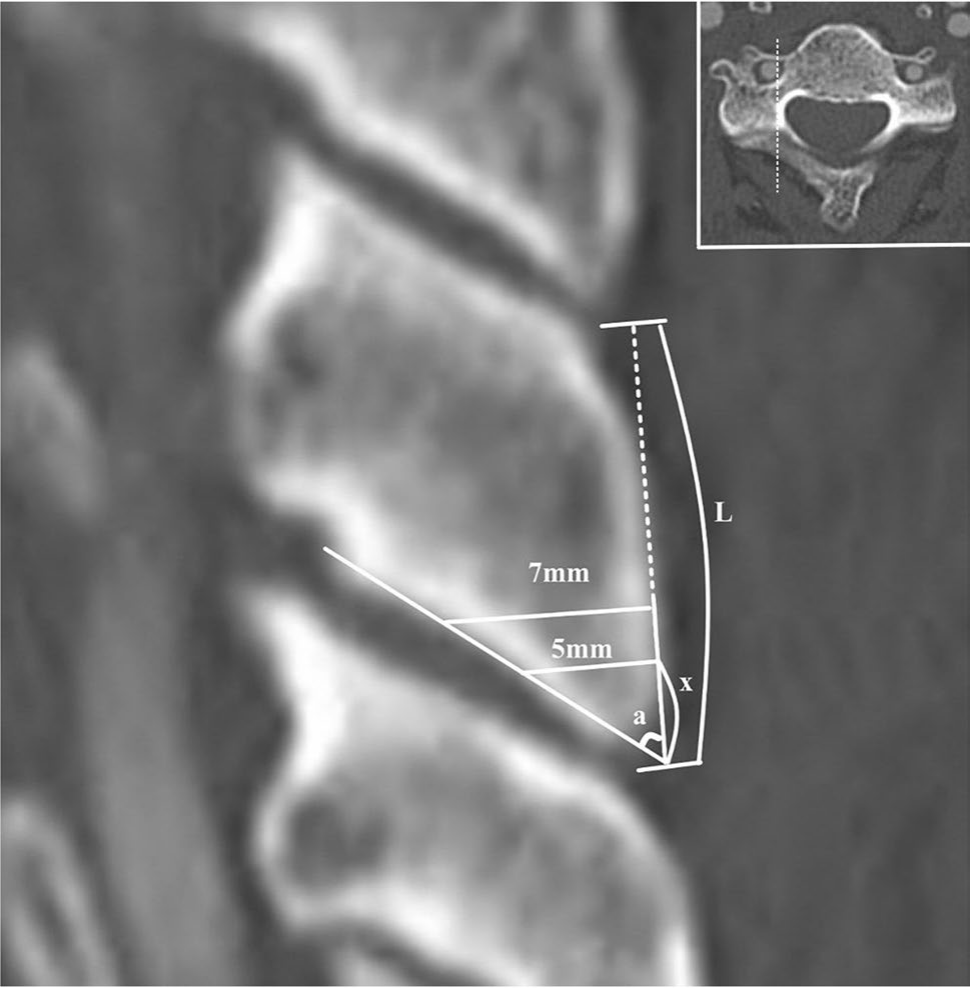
Radiological measurement. The posterior surface length of the lateral mass (L) is measured as the distance between the cranial edge and caudal edge of the lateral mass in sagittal computed tomography images. The inferior pole angle of the lateral mass (a) is measured as the angle between the line drawn through the posterior surface of the lateral mass and the line drawn through the ventral-inferior border of the lateral mass that compose the facet joint. The minimum distance for screw placement (x) is calculated as the inferior pole angle (a) and the length of the screw (5 or 7 mm) using the following equation: [x = length of screw ÷ tangent (a)].
